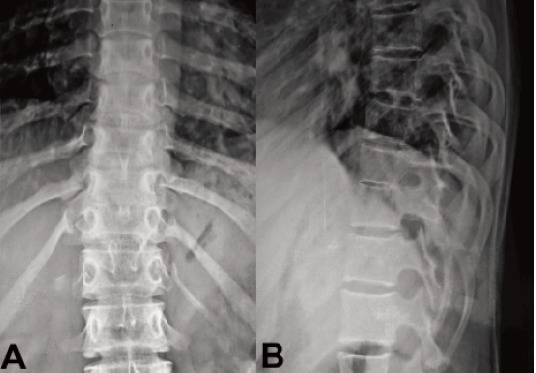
Fig. 2: MRI dorsal spine showing an intraspinal well defined mass lesion extending from D8 to D10 vertebral levels and compressing the spinal cord. The lesion was hypo intense on T1W images and mildly hyper-intense on T2W images. F2:
